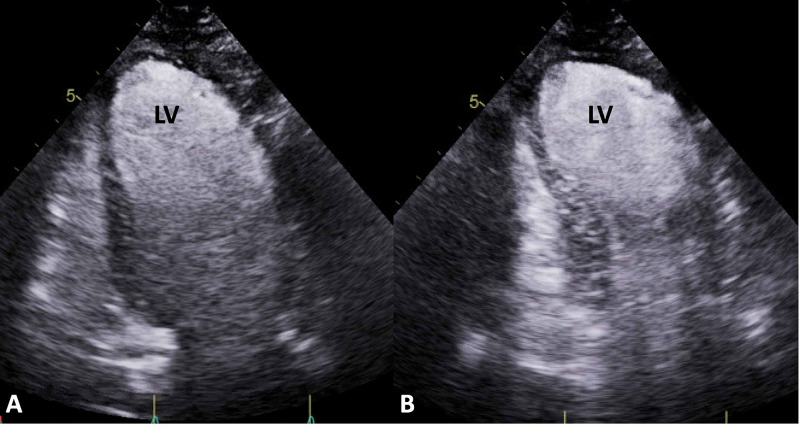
Transthoracic echocardiogram (ECHO). (A) Transthoracic ECHO with contrast in apical two-chamber view during diastole. (B) Transthoracic ECHO with contrast in apical two-chamber view during systole demonstrating left ventricle with apical ballooning and akinesis.
